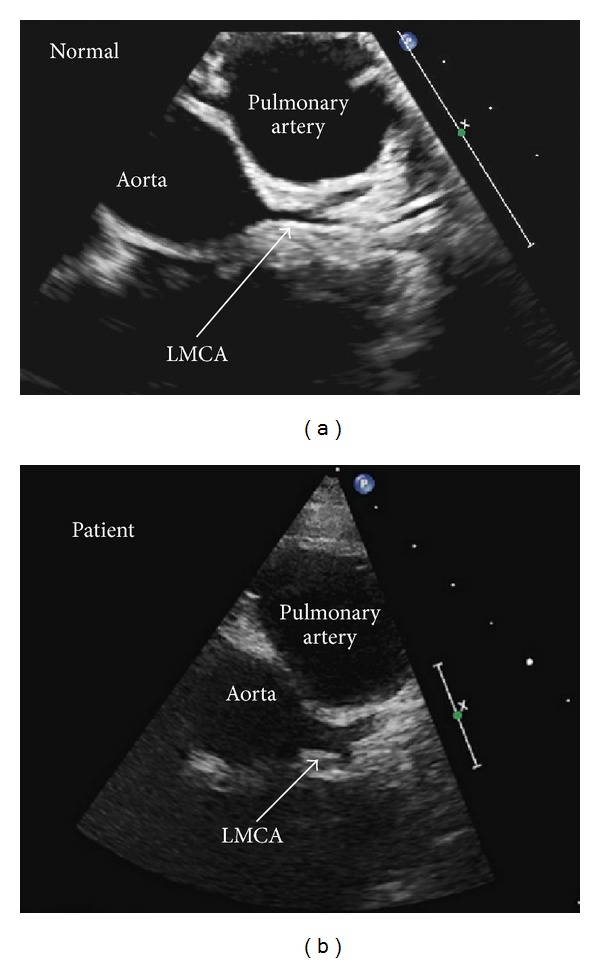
Parasternal short axis view showing dilated LMCA of our patient with normal shown for comparison.
